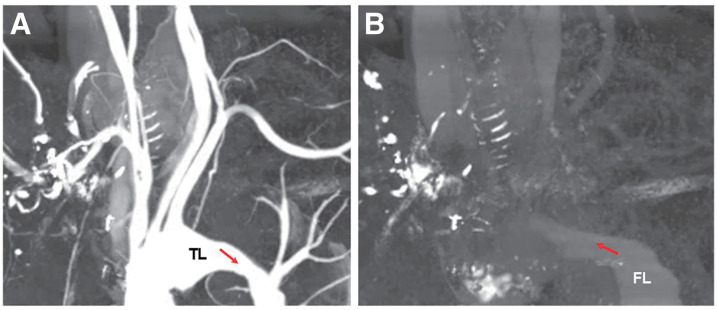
4DCT findings. (A) Early-phase images demonstrated antegrade opacification predominantly within the TL. (B) Delayed-phase images demonstrated retrograde opacification ascending within the FL toward the aortic arch, indicating that the residual FL was not an isolated thrombosed cavity but rather a delayed-flow pathway communicating with the proximal aorta and supra-aortic circulation. TL, true lumen; FL, false lumen; 4DCT, four-dimensional computed tomography
